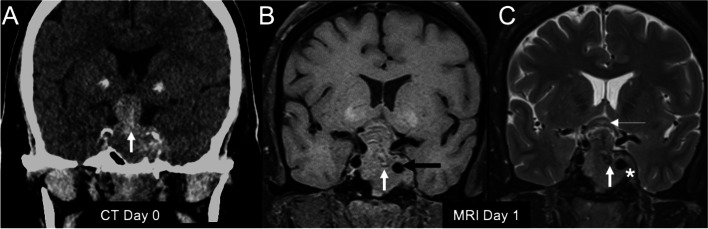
Initial imaging examinations of the 64-year-old man obtained from the outside hospital. A An unenhanced computed tomography image showed the typical “snowman” or “figure of 8” configuration of pituitary macroadenoma with internal hyperdense areas consistent with acute haemorrhage within the tumour (white arrow). B-C This haemorrhagic macroadenoma was confirmed on magnetic resonance images obtained 1 day later. The foci of haemorrhage were isointense to slightly hyperintense (white arrow) on unenhanced T1-weighted imaging (B) and hypointense (white arrow) on T2-weighted imaging (C). The tumour was found to be encasing the normally sized left cavernous internal carotid artery (black arrow in (B)) and invading the left cavernous sinus (asterisk in (C)). C The optic chiasm (thin white arrow) was elevated by the suprasellar part of the tumour
